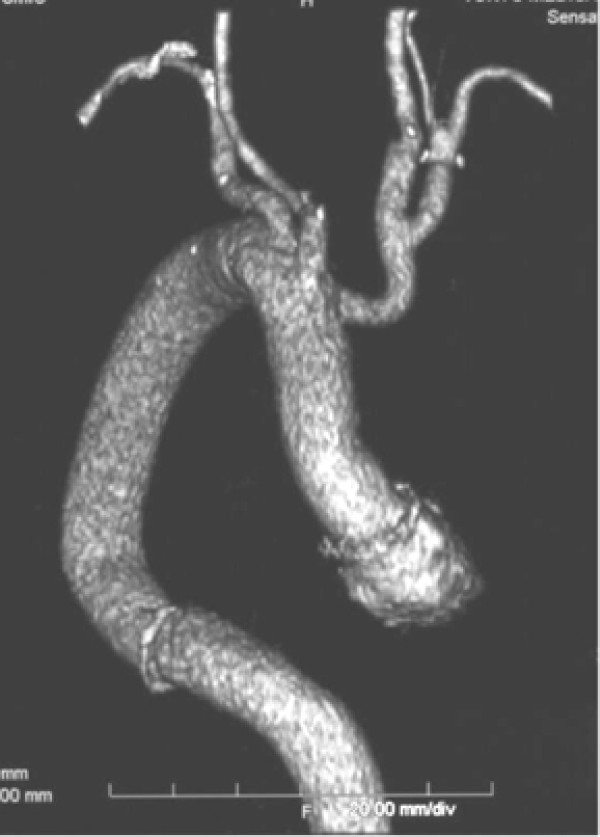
Postoperative 3D CT scan indicating successful repair of the total thoracic aorta
